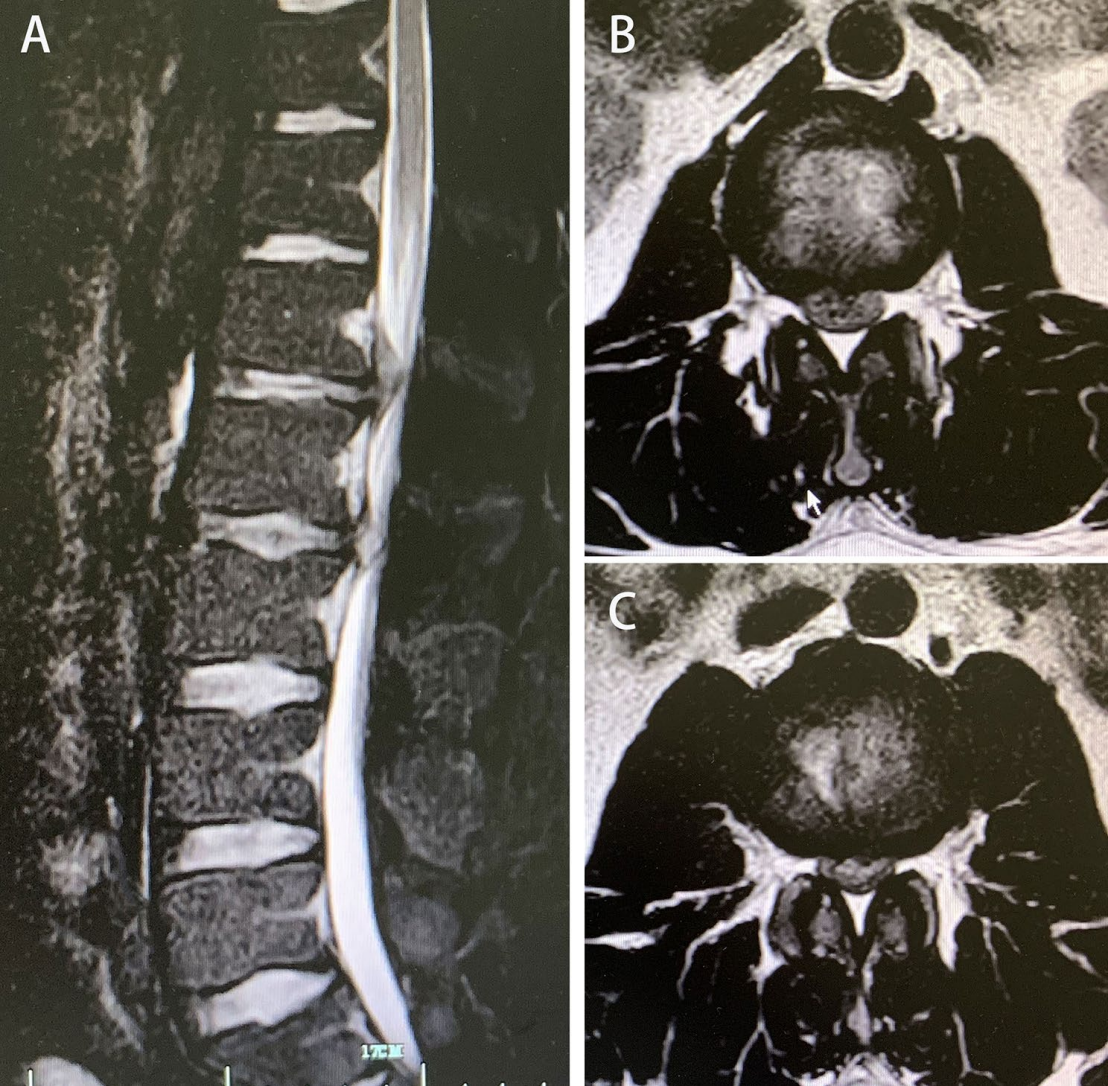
Presurgical magnetic resonance imaging (MRI) showing L1-L2 and L2-L3 disc herniation: (A) Sagittal view, (B) L1-L2, and (C) L2-L3.
